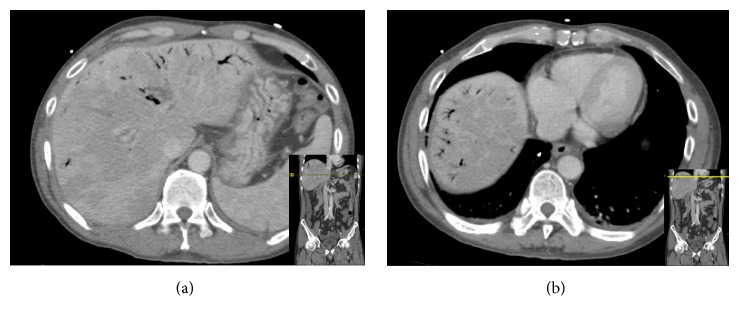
CT scan of the abdomen showing diffuse portal venous gas at different levels of the hepatic parenchyma.
